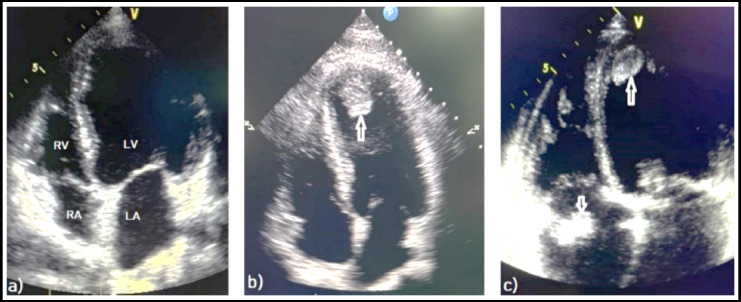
Transthoracic echocardiography images shows a) normal all cardiac chambers b) left ventricular thrombus (arrow), and c) left ventricular thrombus (long arrow) and right atrial thrombus (short arrow). LV, left ventricle; RV, right ventricle; LA, left atrium; RA, right atrium.
